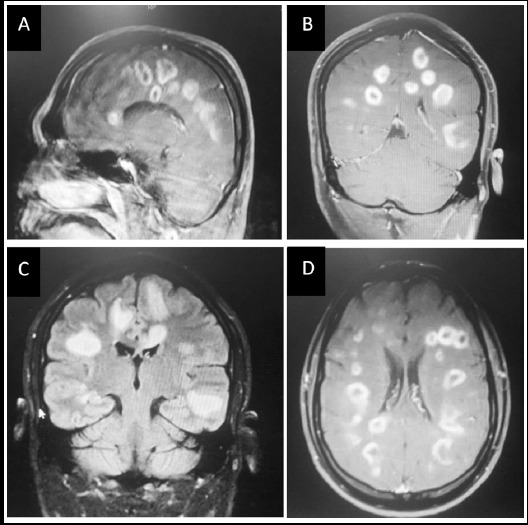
Contrast enhanced MRI T1 weighted images showing multiple ring enhancing lesions in white and grey matter of supratentorial region bilaterally.
